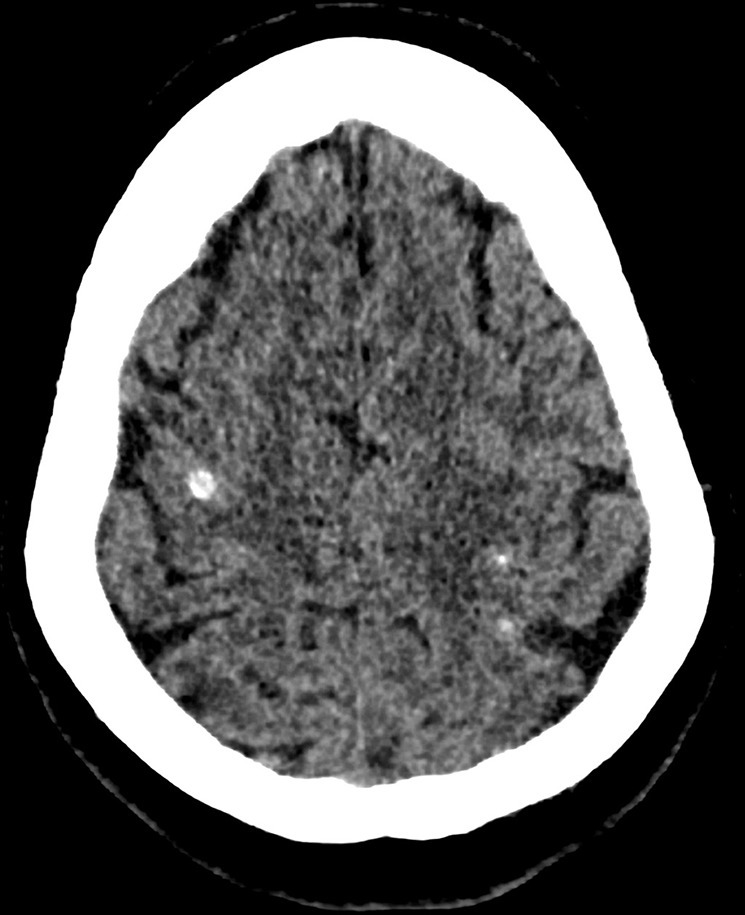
Non-contrast CT brain demonstrating bilateral supratentorial hyperdense punctate haemorrhagic foci. The largest lesion was infratentorial, in the right cerebellar hemisphere (depicted on MRI images).
